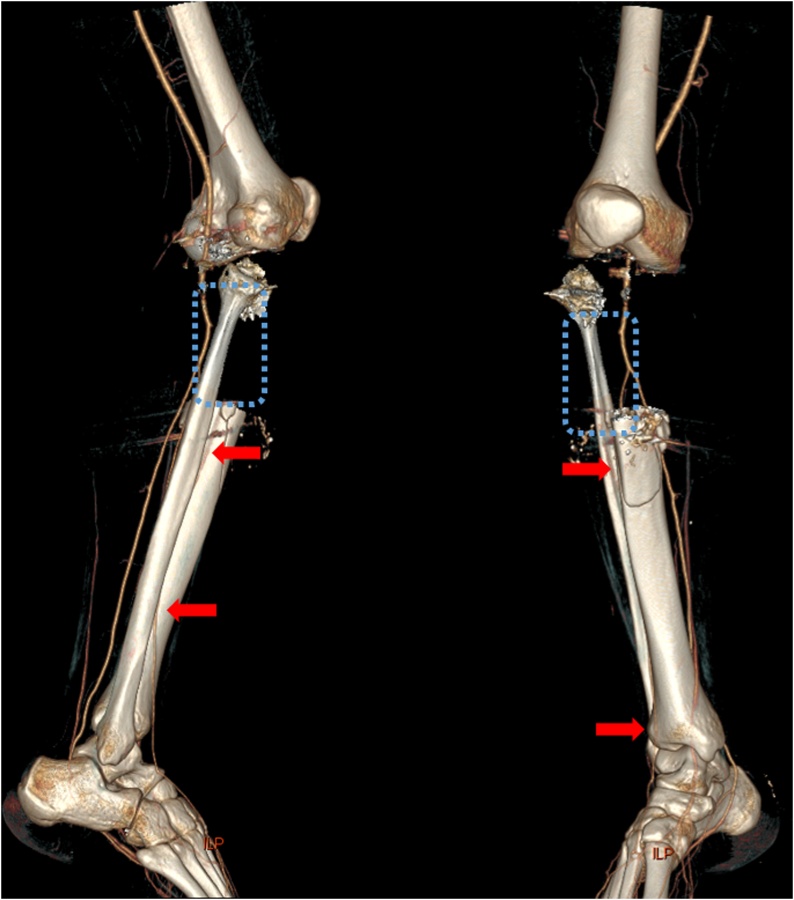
Computed tomography angiography at right knee with the reduction of metallic artifact. (Red arrow: anterior tibia artery with contrast enhancement, Blue dash box: missing segment of anterior tibia artery with poor contrast enhancement).
